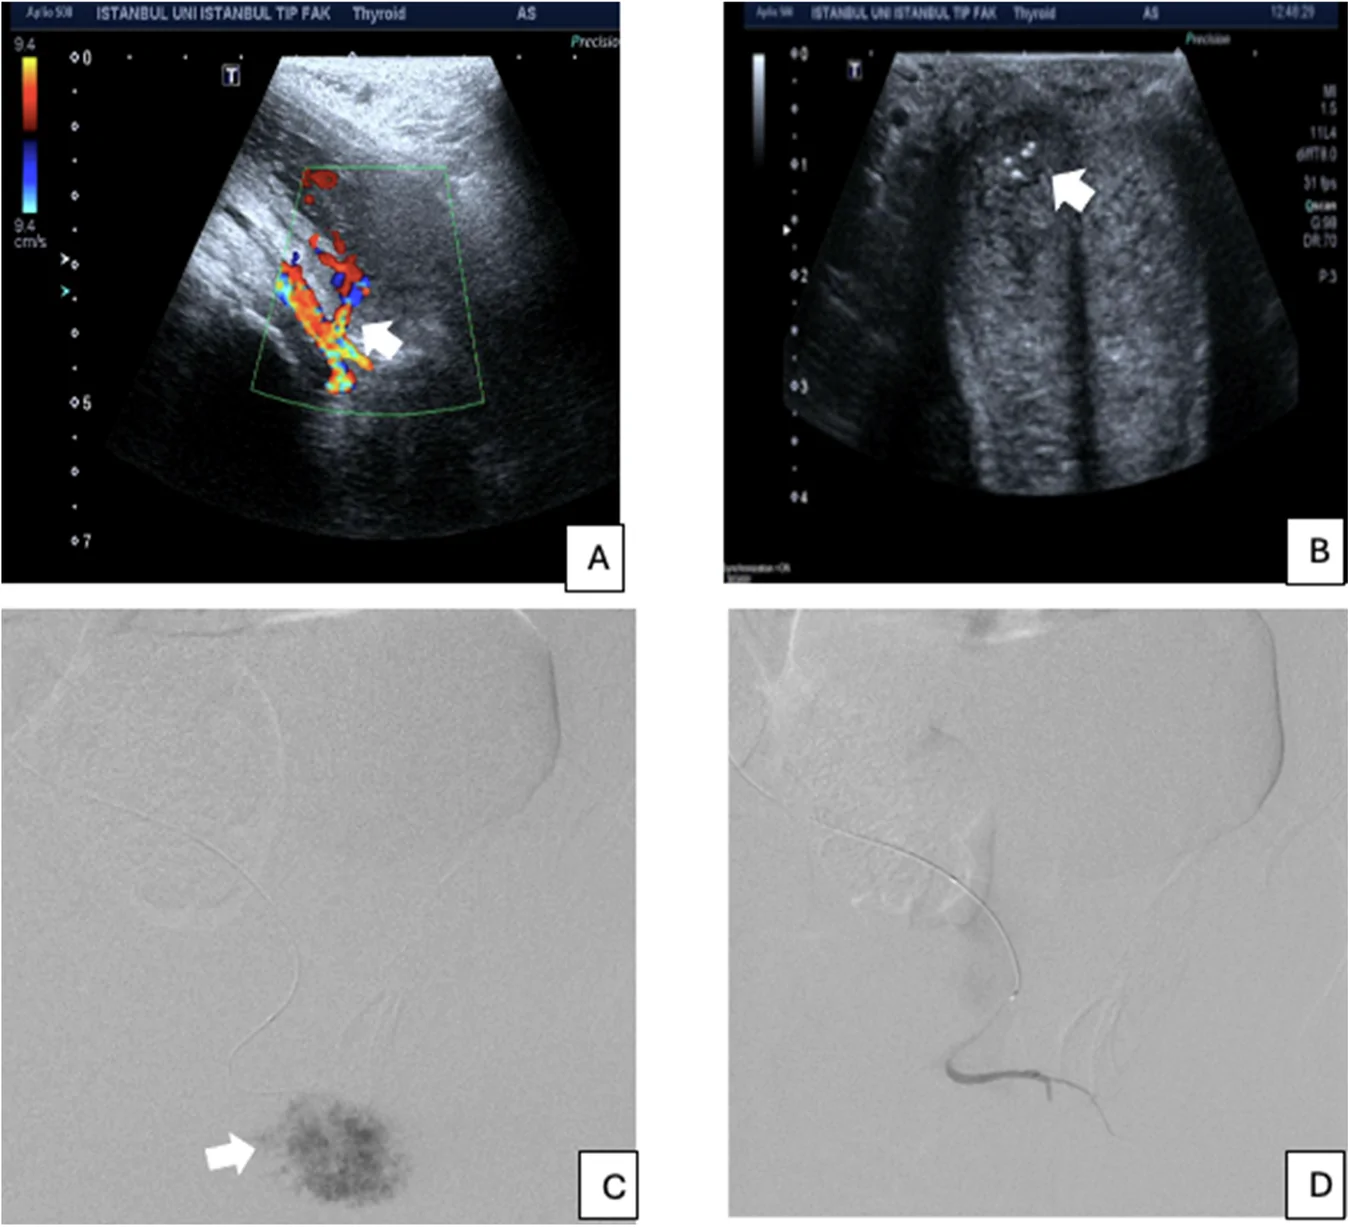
Pre- and post-treatment imaging in a patient with high-flow priapism. A Color Doppler image showing a fistula with turbulent flow (arrow); B Coronal B mode ultrasound image demonstrating no evidence of fistula. Hyperechoic foci (arrow) are seen, representing dispersed gelatin sponge particles within lacunar spaces, a finding associated with embolization in early ultrasound follow-u; C Selective angiogram of the right cavernosal arteries demonstrating an arteriocavernous fistula (arrow); D Selective angiogram after absorbable gelatin sponge embolization demonstrating successful embolization of the arteriocavernous fistula (Archive of İstanbul Faculty of Medicine).
